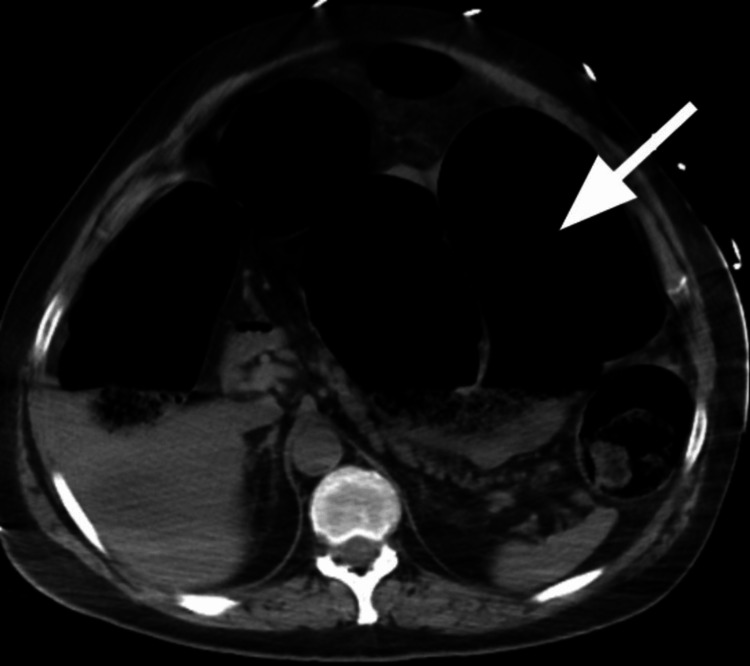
CT abdomen axial view showing massively dilated colon (white arrow).
